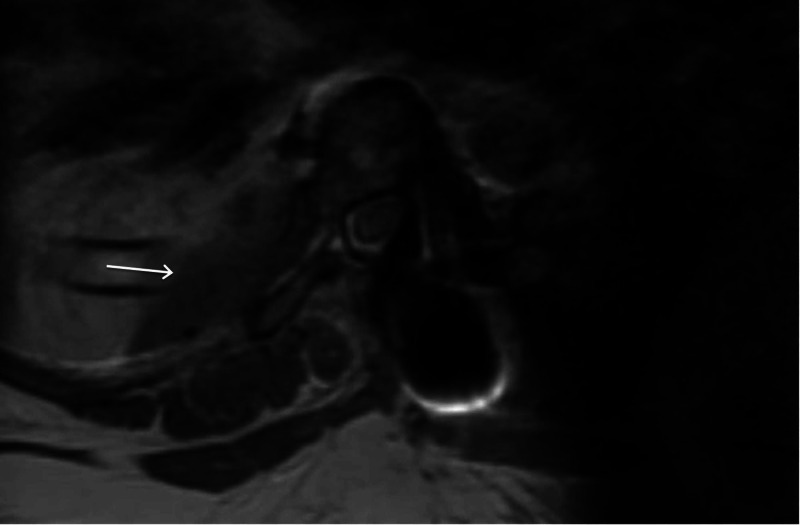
Postoperative MRI of the thoracic spine, contrast-enhanced T2-weighted axial view shows interval resection of previous right T7-T8 paraspinal and neuroforaminal mass with no residual enhancing mass seen. And a small fluid collection identified at the right paraspinal operative site (arrow).
